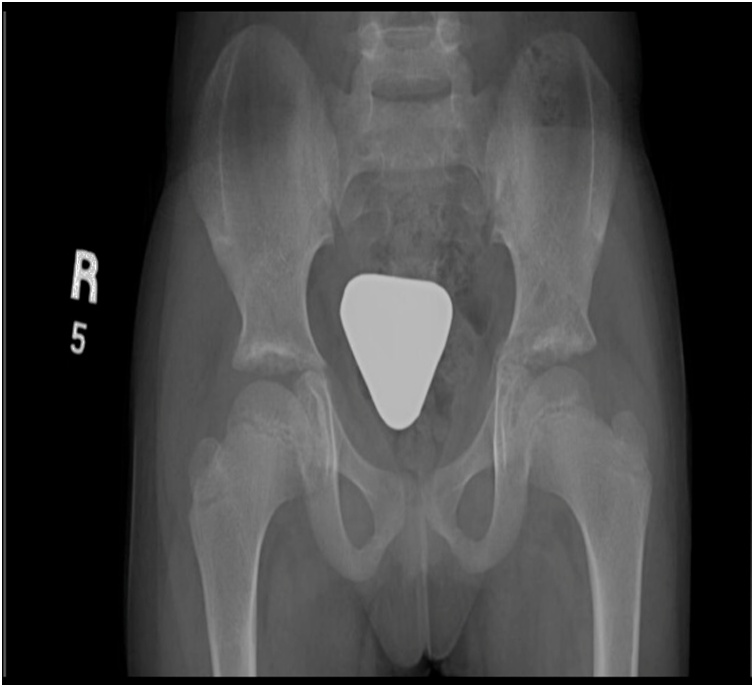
Pelvis AP x-ray at age of 7 years after removal of femur implants.
